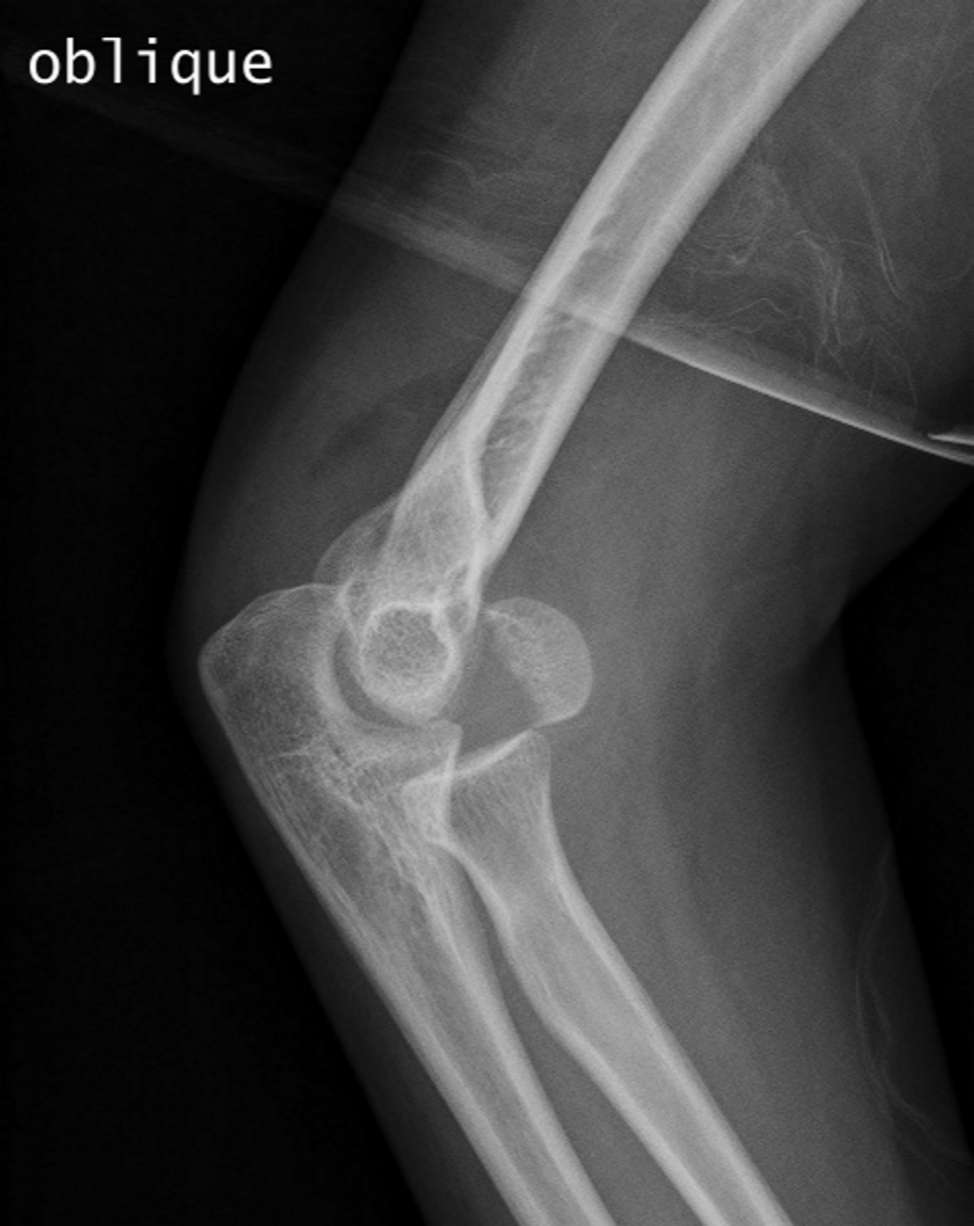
Right Hahn-Steinthal Type 1 capitellum fracture, preoperative oblique radiograph.
